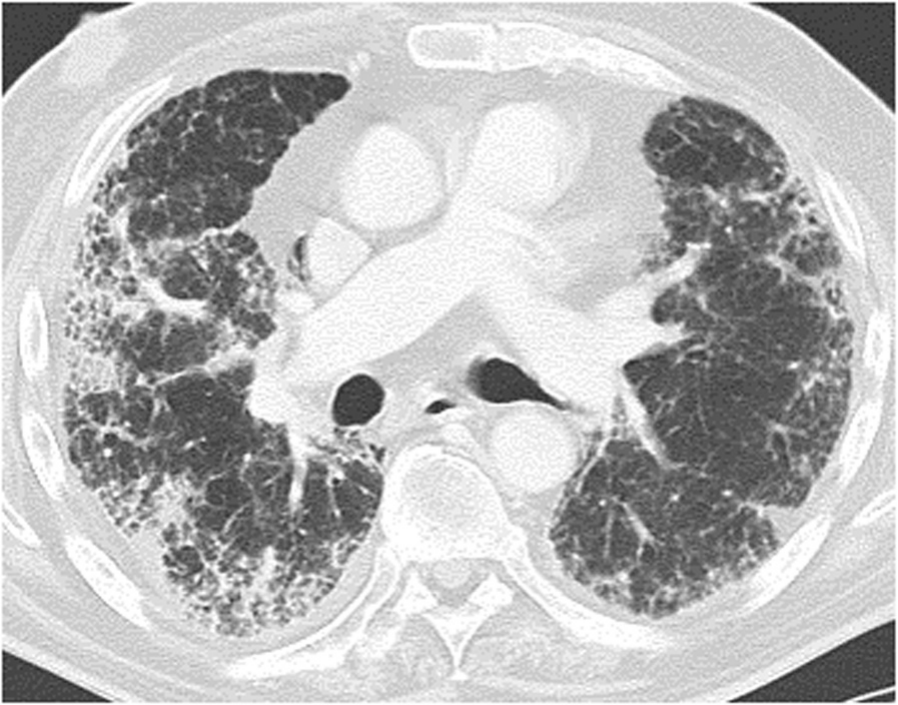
Drug-induced lung disease with a NSIP pattern (non-specific interstitial pneumonia). Patient with chemotherapy for bladder cancer. Chest CT at the level of the right pulmonary artery at lung window. Diffuse bilateral peripheral reticular pattern, ground glass and some consolidation
